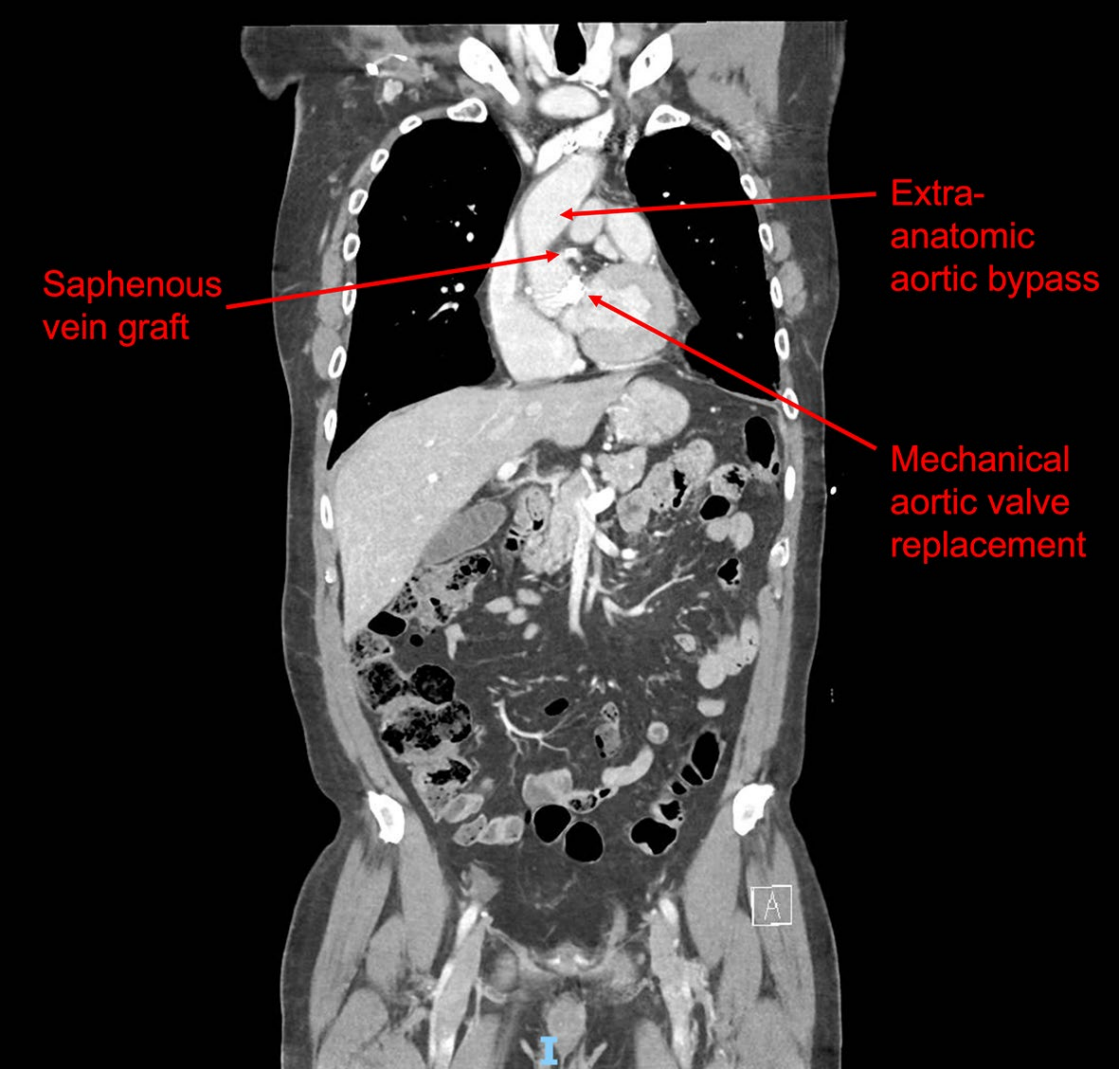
Post-operative coronal computed tomography image of extra-anatomic aortic bypass, saphenous vein grafts, and mechanical aortic valve replacement
